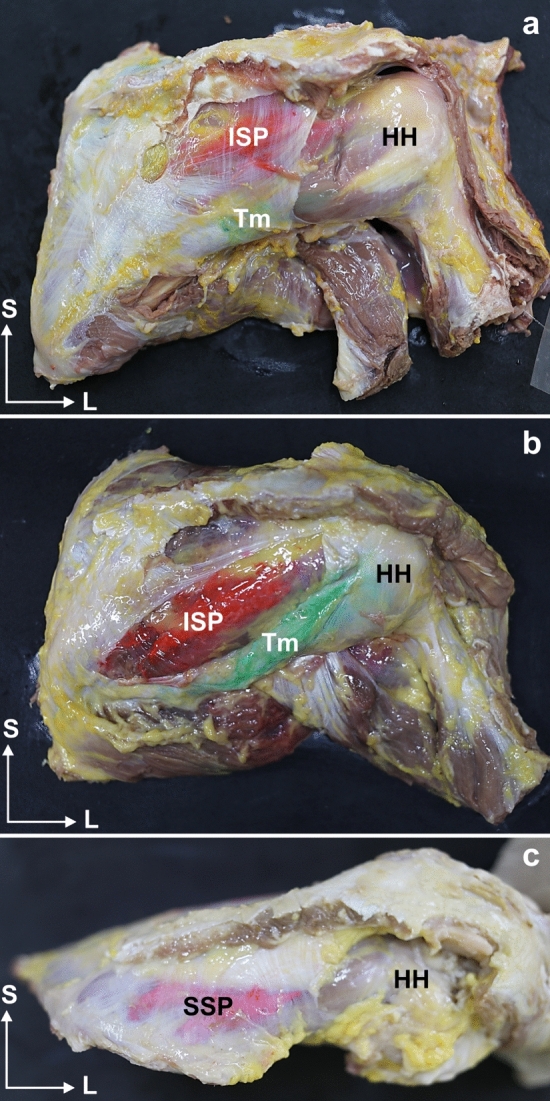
Anatomical view of the posterior side of the shoulder. a The muscular fascia covers the muscles. b The muscular fascia is removed. It is possible to see the red dye injected by the ultrasound guide only at the infraspinatus and the green dye only at the Tm muscle. c Superior view of the shoulder. The muscular fascia forms an encapsulation of the supraspinatus muscle, and the red dye injected keeps down the muscular fascia. Abbreviations *ISP*: infraspinatus, *Tm:* teres minor, *SSP*: supraspinatus, *HH:* humeral head, *S*: superior, *L*: lateral
